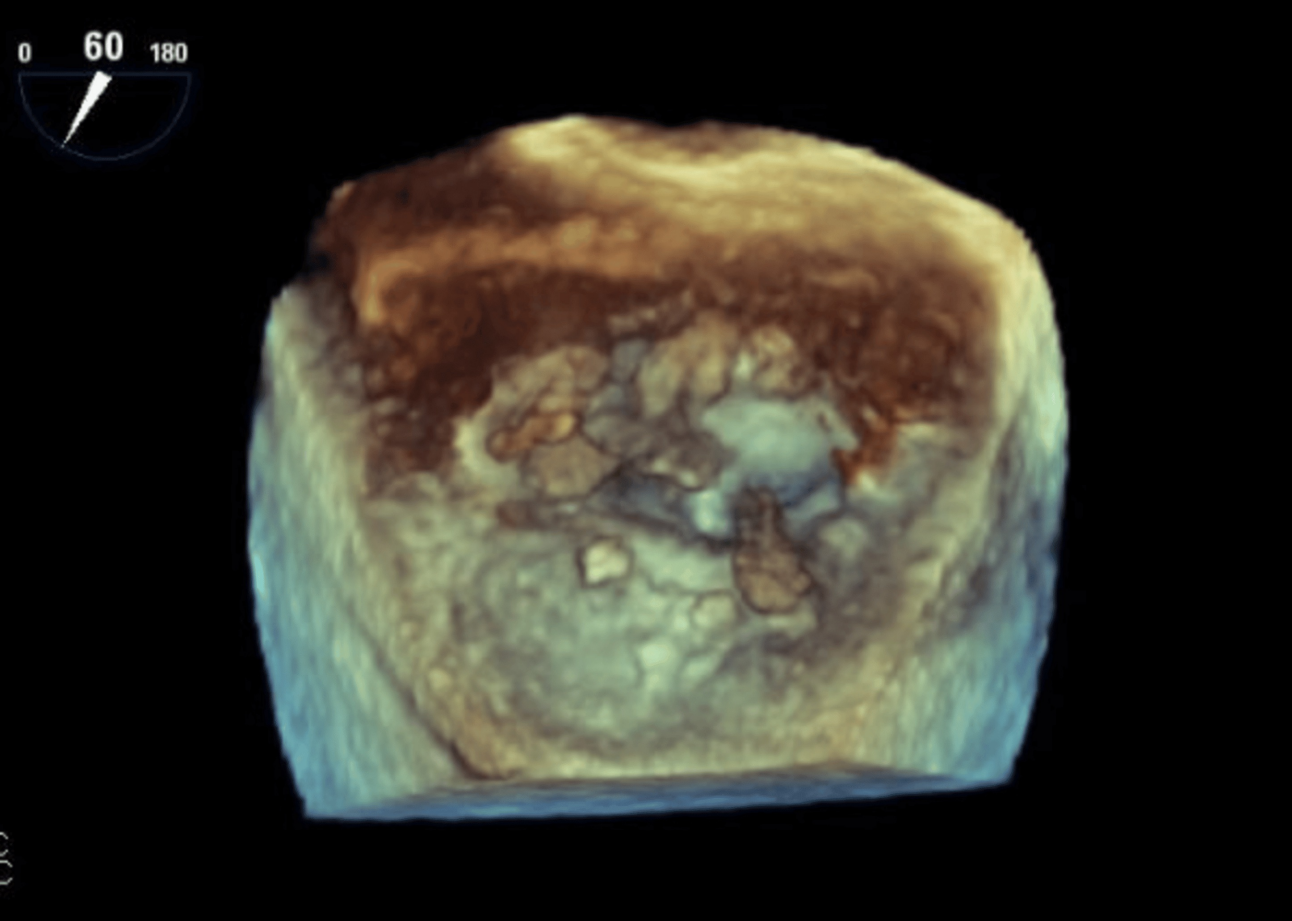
Transesophageal Echocardiography (TEE) of the Prosthetic Mitral Valve TEE images showing echogenic structures attached to the atrial aspect of the anterior and posterior mitral leaflets, concerning for thrombus or vegetation. Image was obtained from the patient with their permission.
